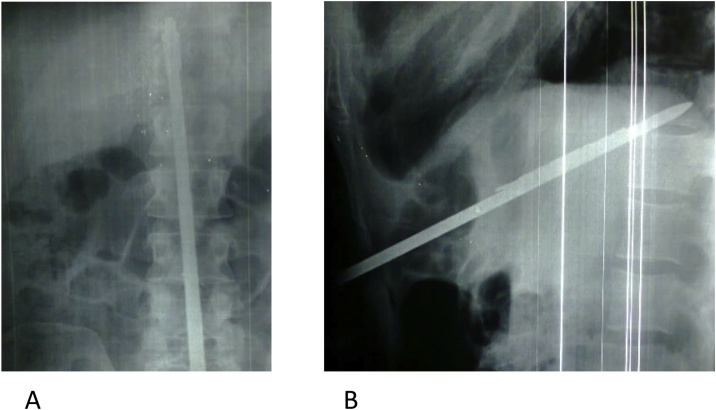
abdominal radiograph (A: face, B: profil) showing the harpoon entry in sub xyphoid, ending in the D11 vertebral body.
